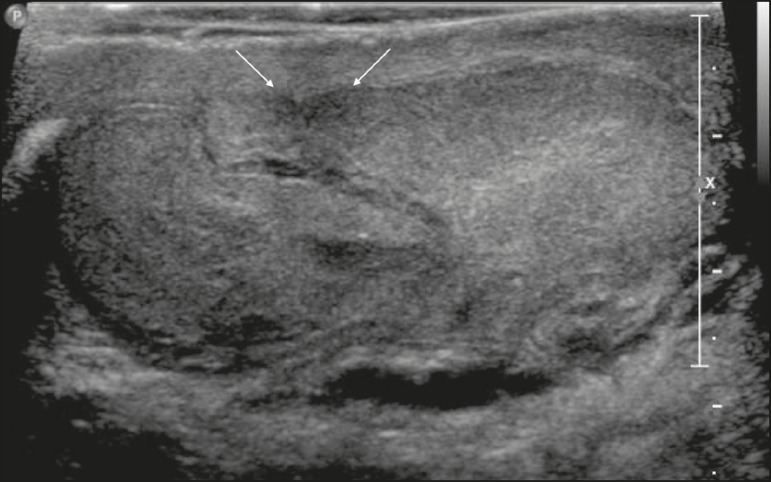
Testicular trauma. Post-trauma ultrasound of the testis showing a
